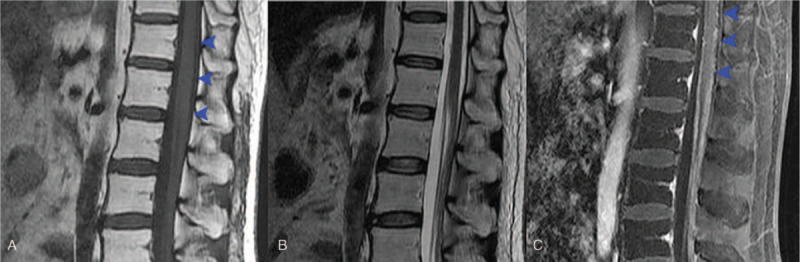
Spinal MRI revealed diffuse thickening of the dura matter and multiple tuberous lesion, which were enhanced after gadolinium administration (panel A: T1-weighted, panel B: T2-weighted, panel C: gadolinium contrast enhanced T1-weighted).
